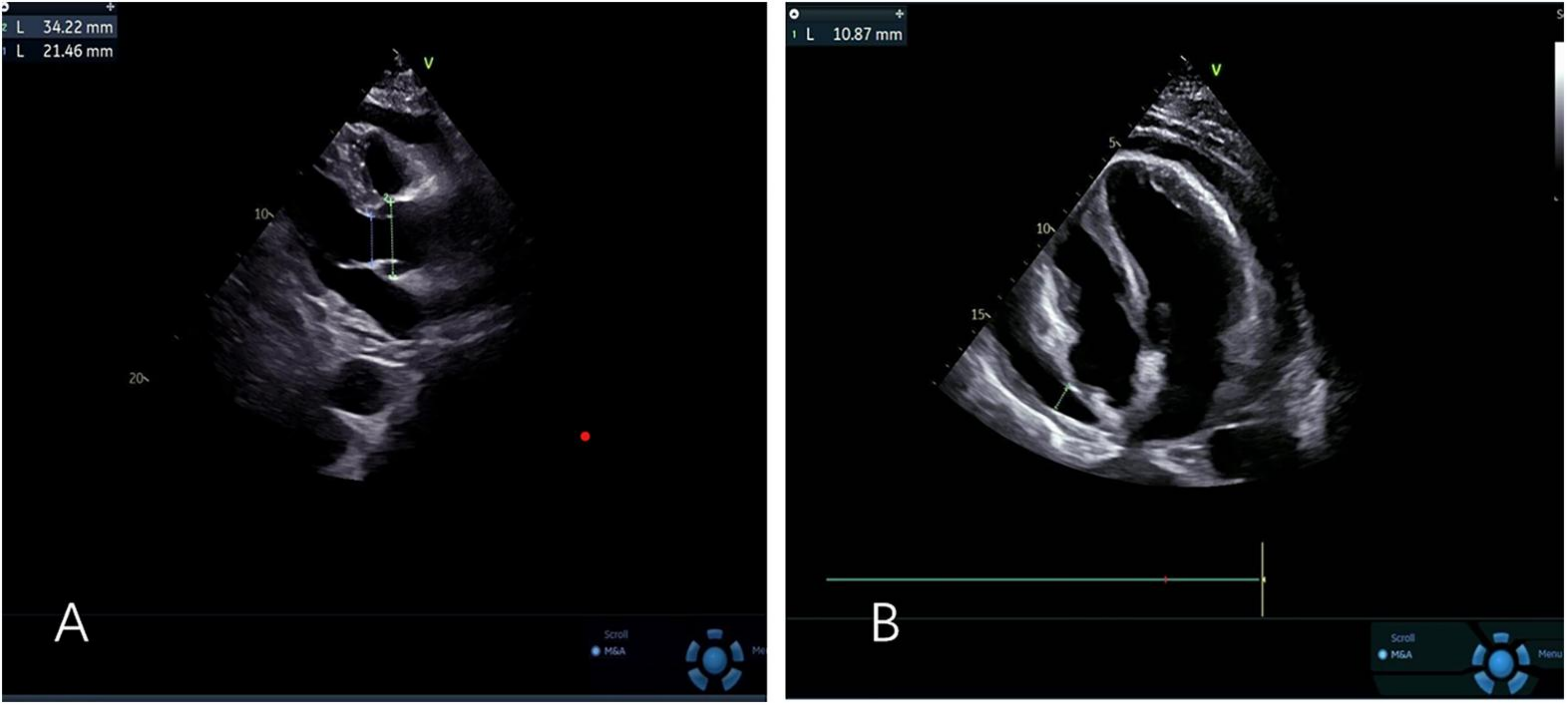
(A) Left ventricular long-axis view reveals minimal pericardial effusion (B) apical four-chamber view demonstrates mild to moderate pericardial effusion.
